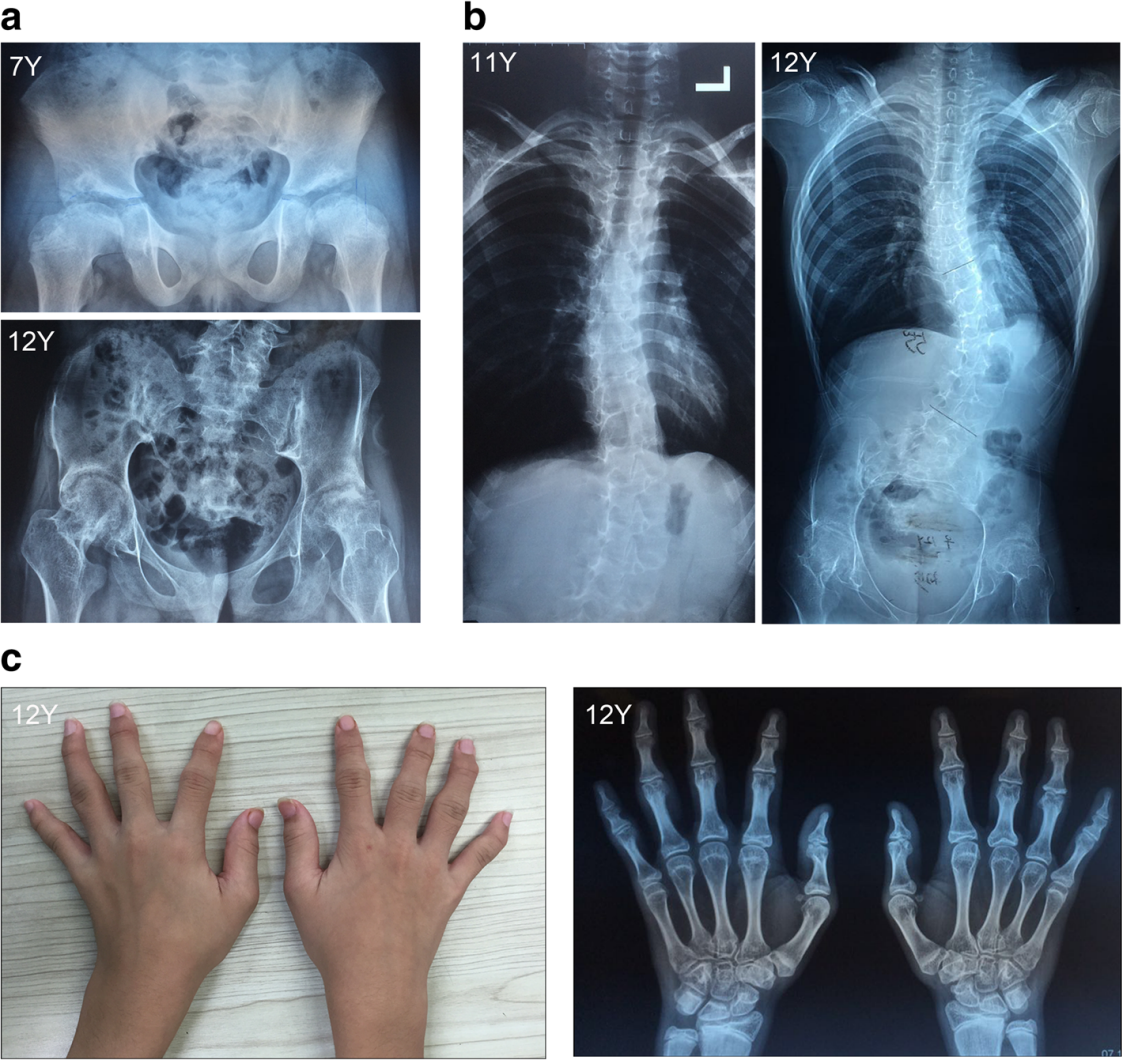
Radiographs and clinical features of patient 1. Hip radiographs revealed coxa plana at the ages of 7 and 12 years (a). Spinal radiographs confirmed the progress of scoliosis (b). Hand radiographs confirmed the brachydactyly and significantly flattened articular surface (c–d)
